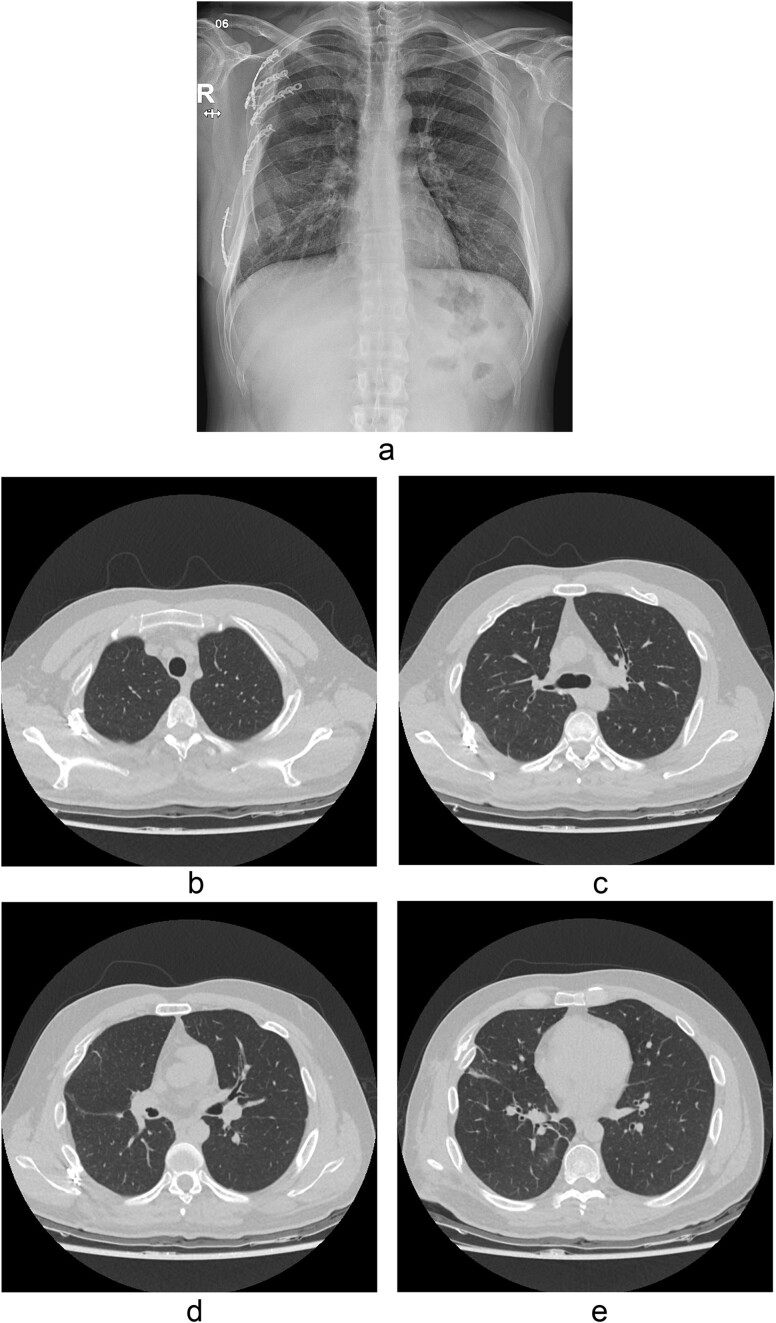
Chest radiograph and CT scan 10 months after the accident. (a) Chest radiograph showed well-healed fractured ribs and expanded lungs without active lesions. (b)–(e) Non-enhanced axial chest CT images showed recovery from injury.
